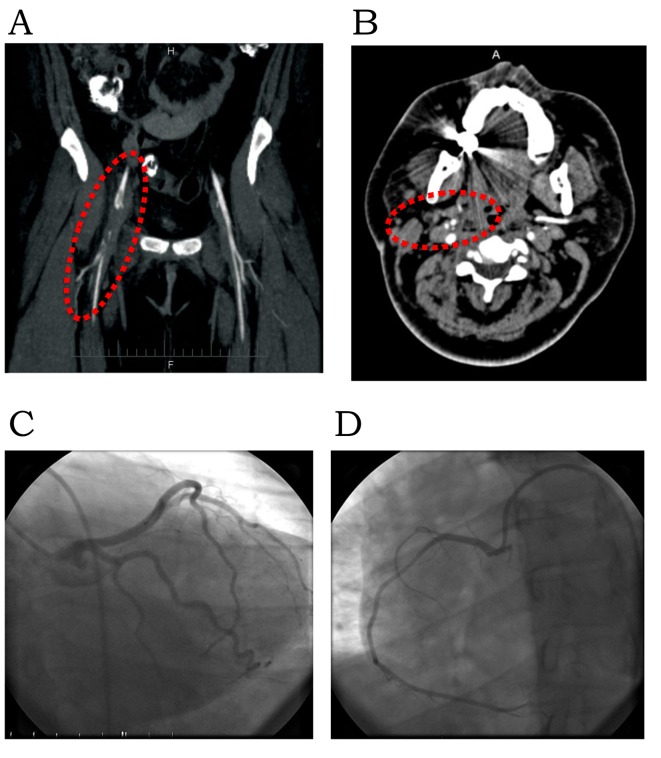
Paradoxical thromboembolism. (A) CT angiogram of lower extremities with emboli (filling defects; dotted circle) in the right illio-femoral arteries. (B) CT angiogram of upper extremities with emboli (filling defects; dotted circle) in the right subclavian artery. Cardiac catheterization, (C) left coronary artery; (D) right coronary artery.
